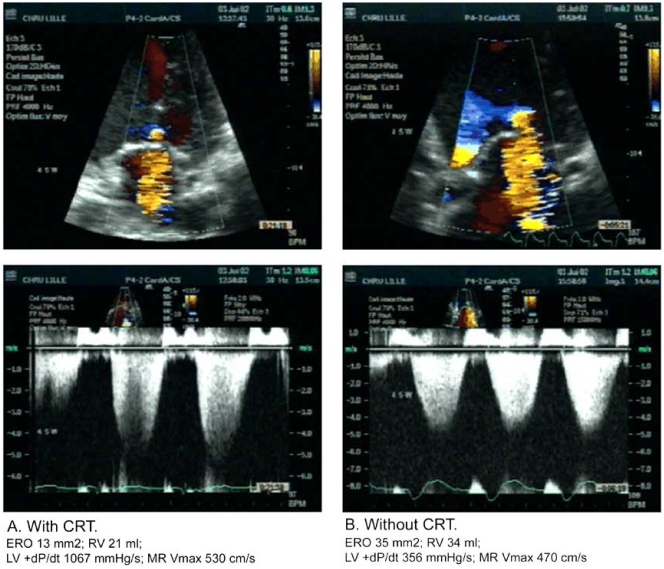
Colour flow (top) and continuous wave (bottom) Doppler echocardiographic recordings in a representative patient at maximal exercise with (Panel A) and without (Panel B) cardiac resynchronisation therapy (CRT). Left ventricular (LV) dP/dt and maximum mitral regurgitation velocity (MR Vmax) were reduced without CRT. ERO, effective regurgitant orifice area; RV, regurgitant flow volume. From Ennezat PV, Gal B, Kouakam C, *et al*. Cardiac resynchronisation therapy reduces functional mitral regurgitation during dynamic exercise in patients with chronic heart failure: an acute echocardiographic study. Heart (British Cardiac Society) 2006; 92(8): 1091-1095.
